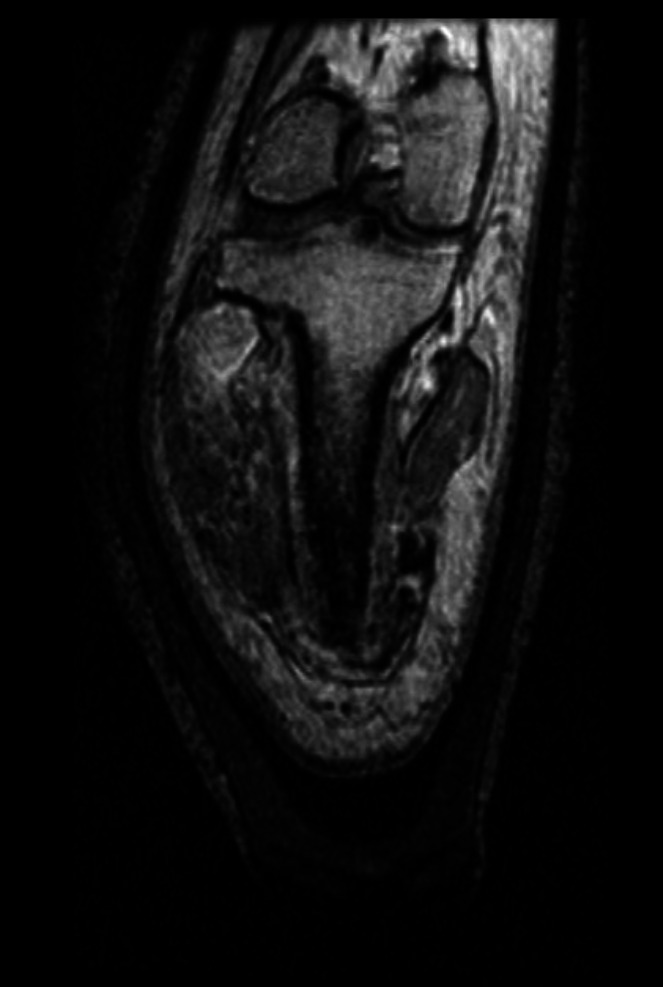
MRI Image in coronal plane of participants residual limb showing femur, tibia, and soft tissues.
